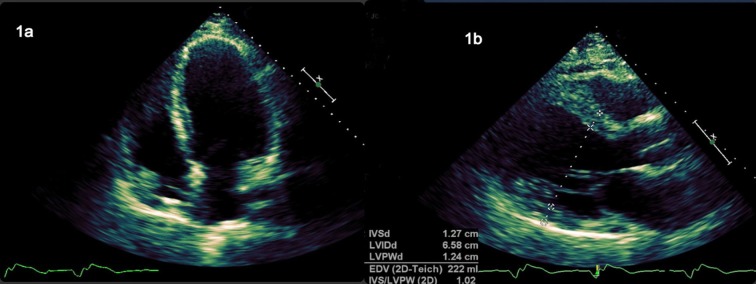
Transthoracic echocardiogram (left parasternal and apical four-chamber views) showing dilated cardiomyopathy with an interventricular thickness of 1.27 cm and an ejection fraction of 25%
